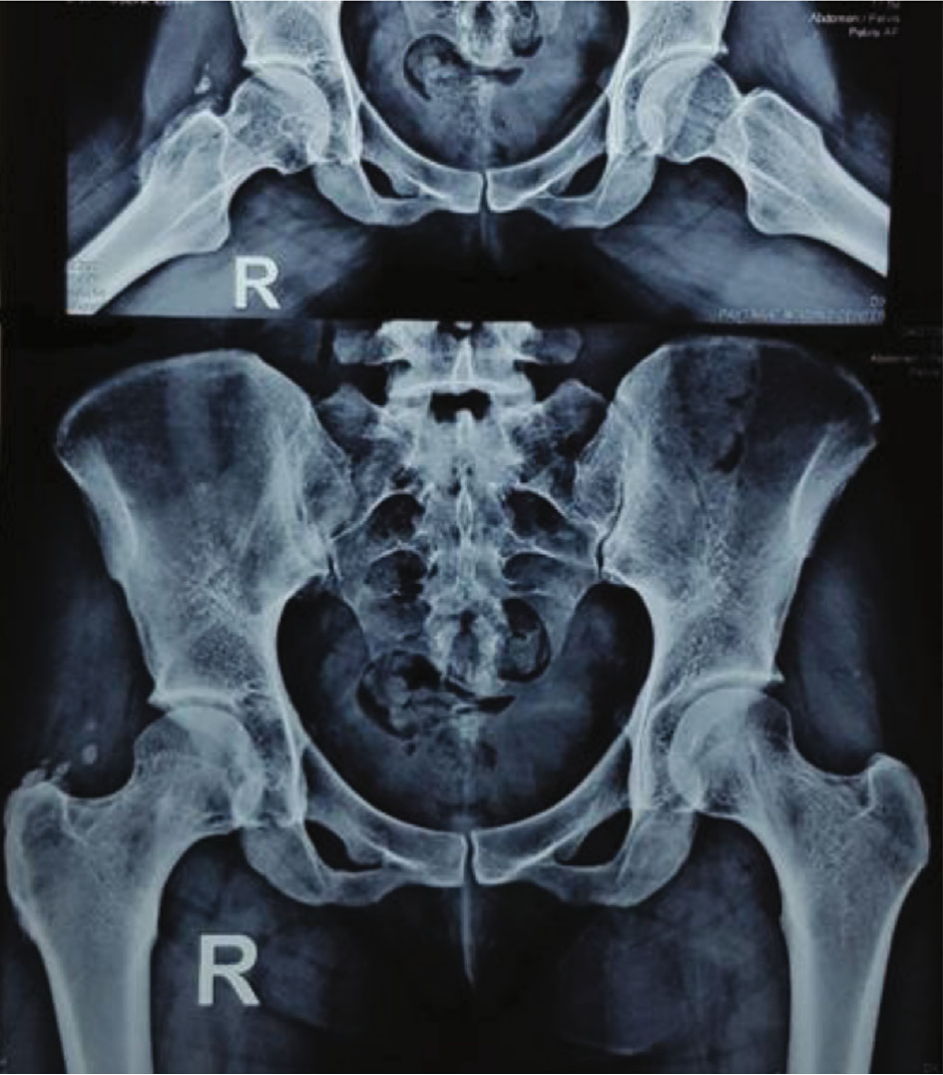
Latest postoperative follow-up X-ray supporting disease-free condition of the right hip.
